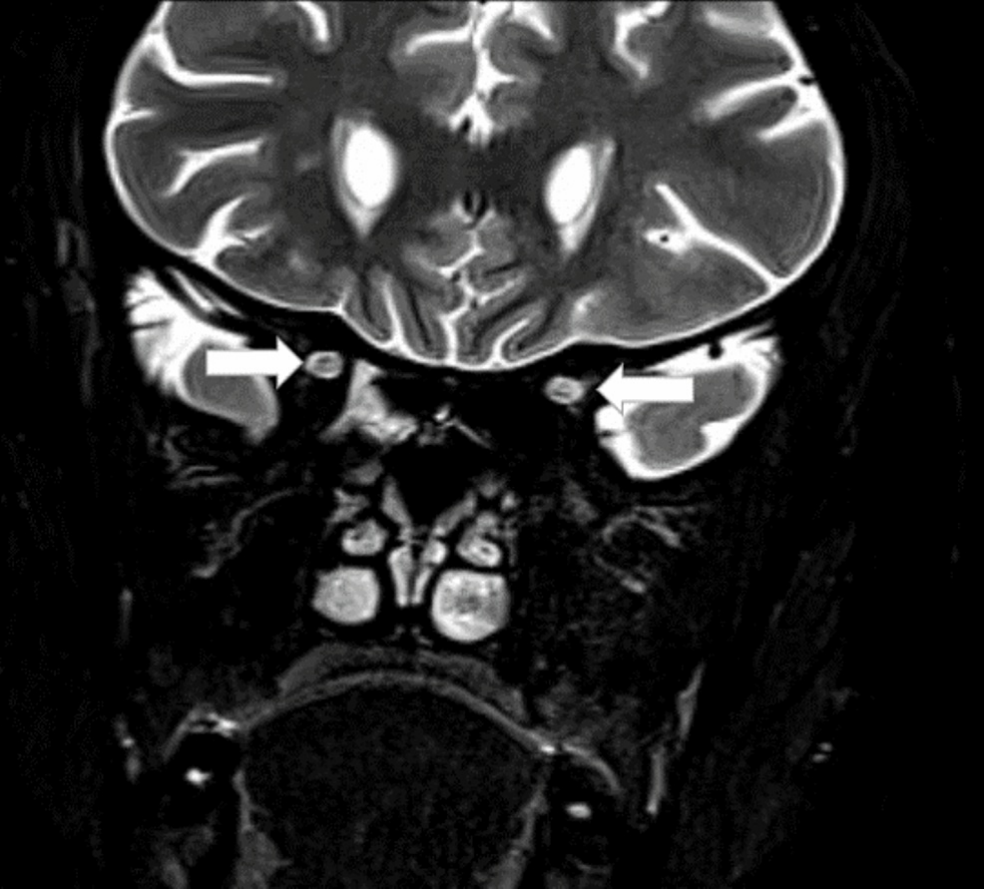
Coronal T2-weighted image of MRI orbit White arrows show hyperintensities in the posterior aspect of bilateral optic nerves.
